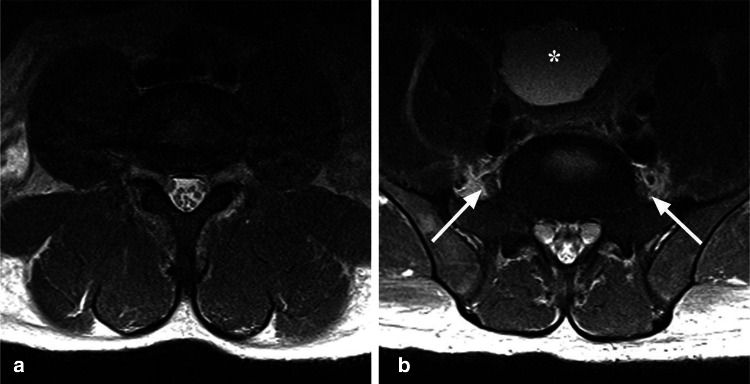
Axial T2-weighted MR images of the lumbosacral spine (a, b), demonstrating mild hypertrophy of the spinal nerve roots. Mild prominence of the lumbosacral plexus bilaterally is also shown (*arrows*). Note the distended urinary bladder with a trabeculated wall (*asterisk*)
